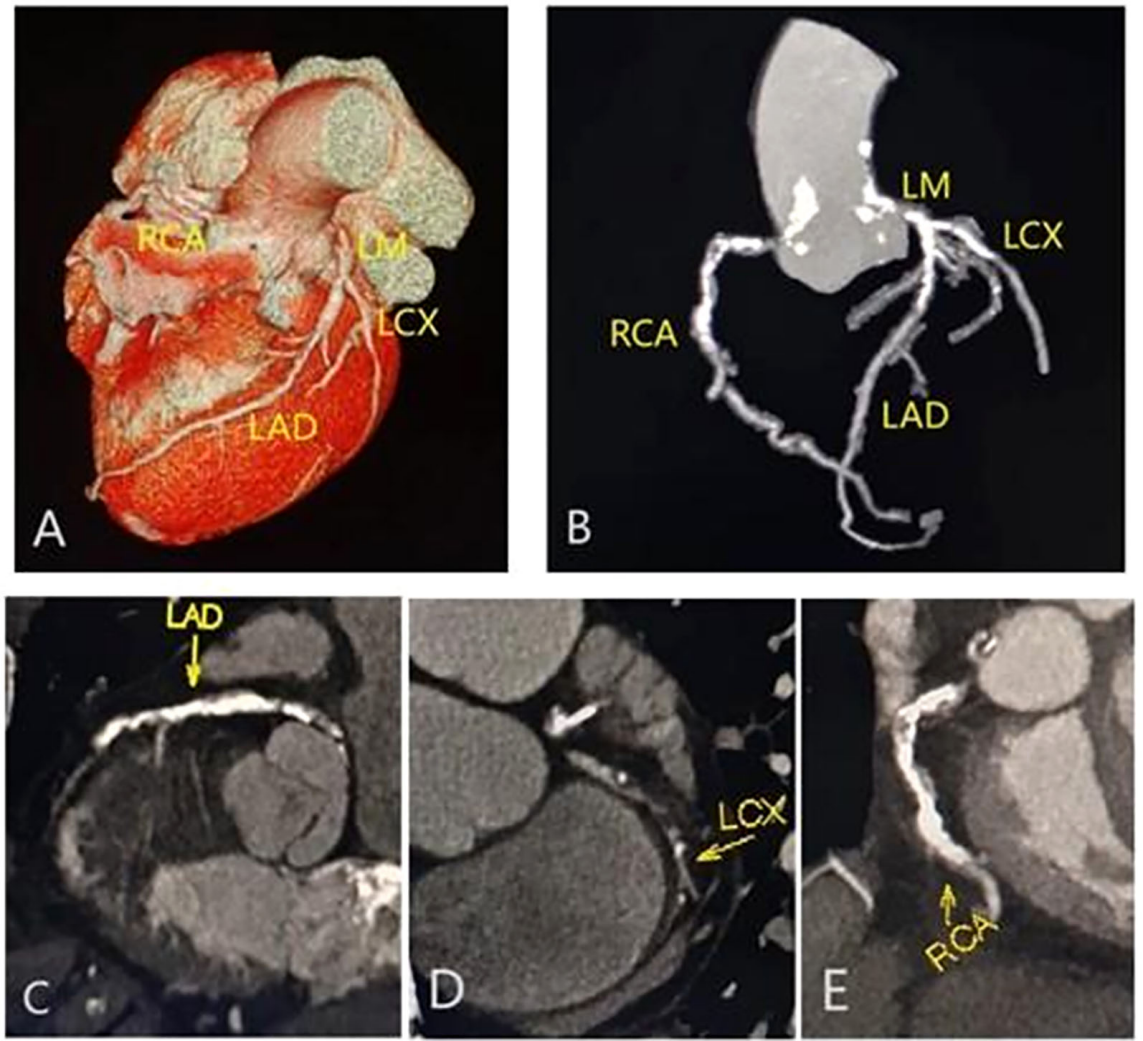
Representative CCTA images of multivessel disease in a male with type 2 diabetes mellitus and metabolic syndrome. Volume rendering image (A); maximum intensity projection (MIP) CT image (B); and curvature plane reconstruction images (C-E) show the non-smooth edges, diffuse calcified, and mixed plaques of the LM, LAD, RCA and LCX.
